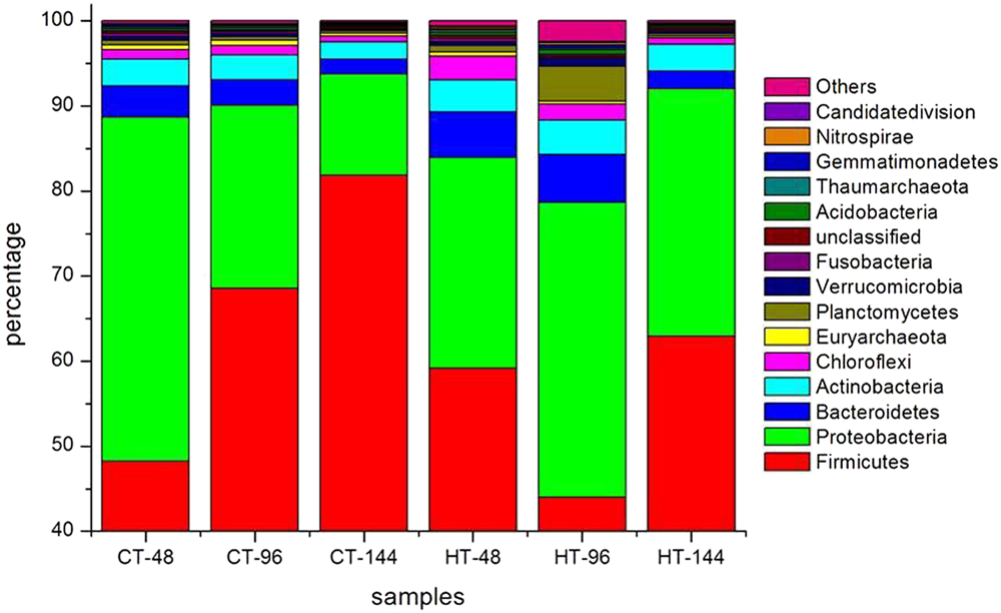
The dominant phyla percentage for all samples at each time point. CT/HT-N is sample mentioned in Table 1.
